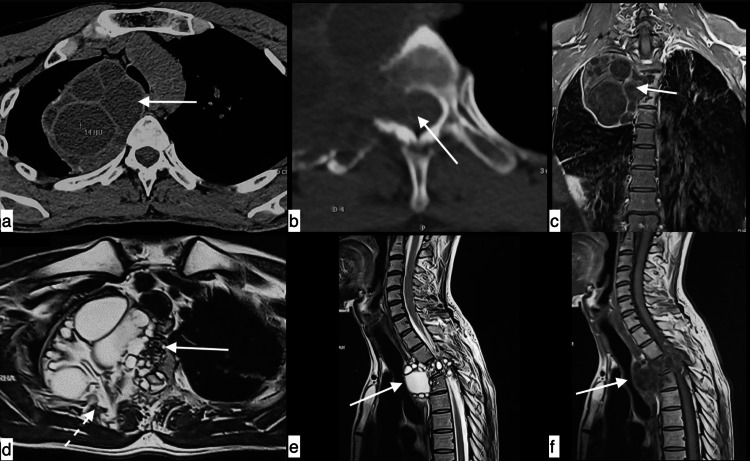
Spinal hydatid cyst A 36-year-old male with a spinal hydatid cyst presenting with cough, fever, and back pain, Axial plain CT (a and b) and coronal (c), axial (d), and sagittal MRI (e and f) showing lytic lesions with well-defined cystic lesions with daughter cysts in spinous process, right transverse process and body of D3, D4 vertebral bodies. The lesion is scalloping the posterior end of the second rib on the right side (dotted arrow) causing bone expansion and projecting into the canal with narrowing and impingement of the cord.
